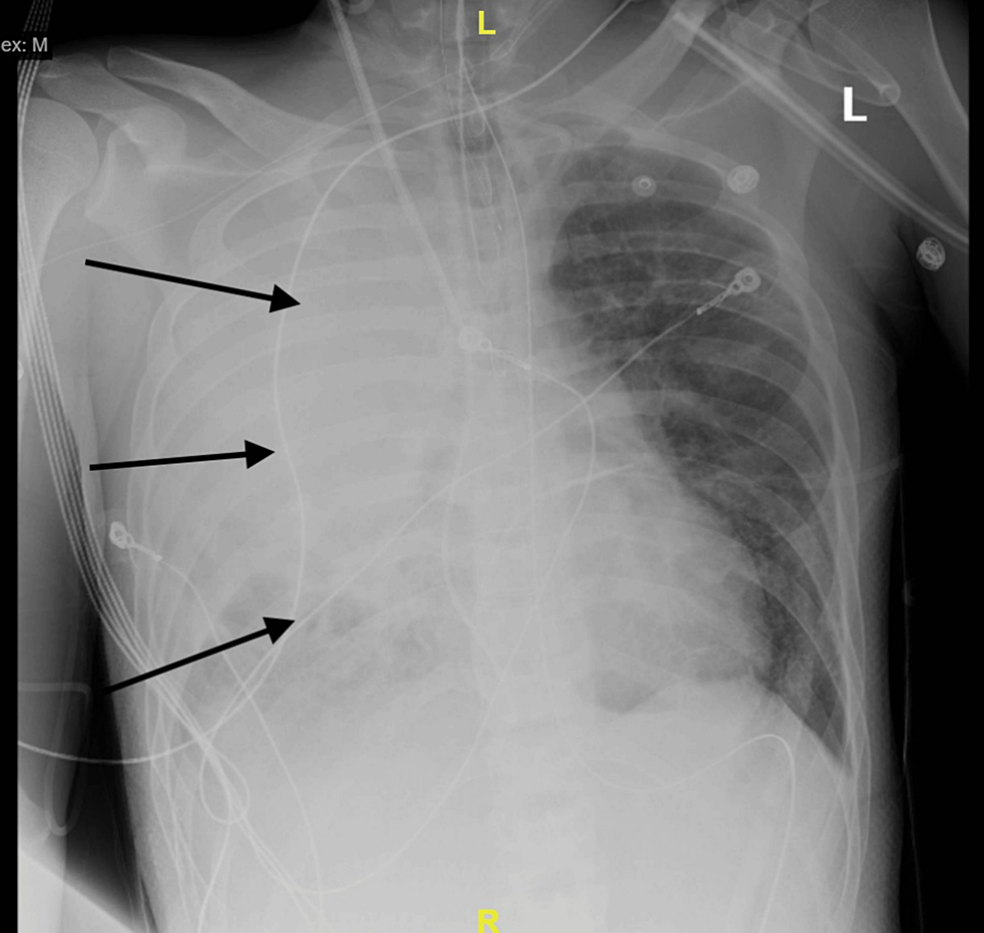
Chest radiography showing worsening right-sided infiltrates
